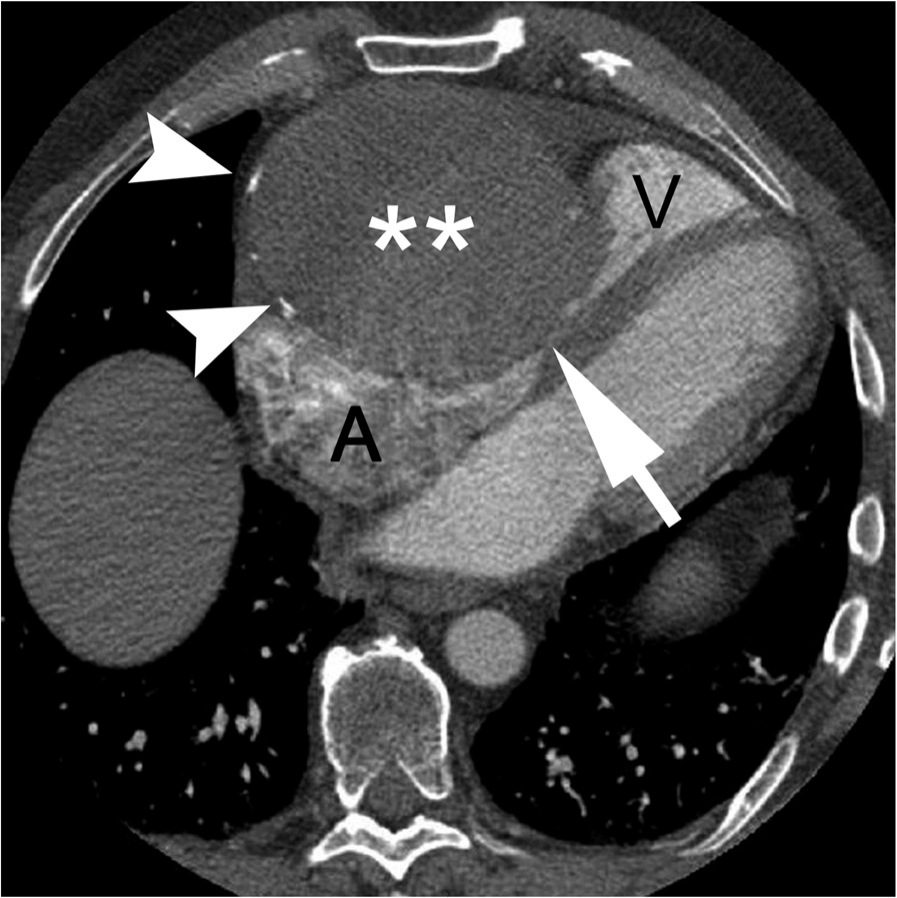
Axial image from CTA. There is a large mass () with mural calcifications (arrowheads). There is marked compression of the tricuspid valve and mid-heart regions (arrow) between the right atrium (A) and right ventricle (V).
